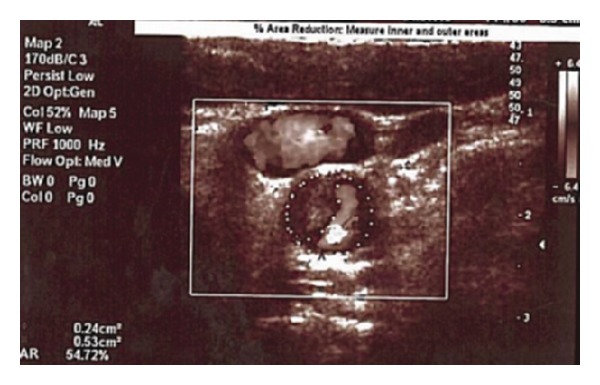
Carotid doppler ultrasonography shows left carotid artery with thrombus formation.
